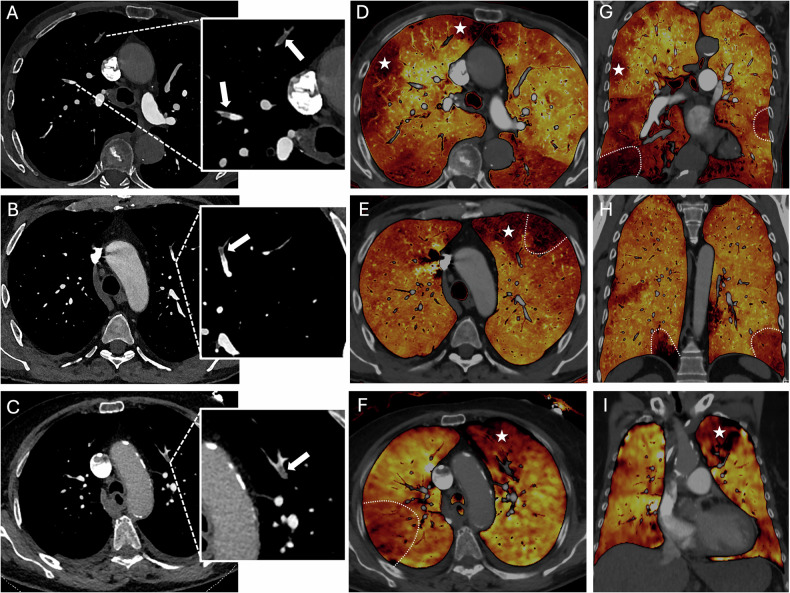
Multifocal peripheral pulmonary embolism. Axial images of UHR-PCD-CTPA at IQ50 (A) and IQ25 (B) vs. EID-CTPA (C) showing peripheral pulmonary embolism (magnified boxes, arrows) with consecutive perfusion deficits (asterisks) in the corresponding axial (D–F) and coronal (G–I) iodine maps. Perfusion deficits resulting from additional pulmonary emboli not depicted in the selected representative images are marked with dashed bows. Note the excellent spatial resolution of CTPA images and iodine maps with PCD in UHR mode, irrespective of IQ level. CTPA, CT pulmonary angiography; EID, energy-integrating detector; IQ, image quality index; PCD, photon-counting detector; UHR, ultra-high resolution
